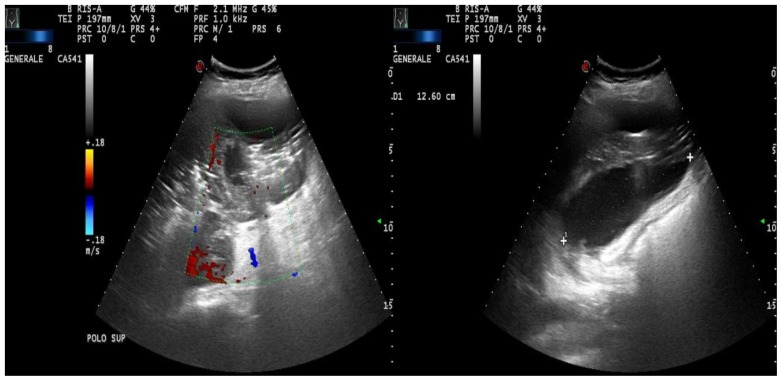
Abdomen ultrasound showing a decrease of the spleen volume and avascularization of the spleen after SSAE.
